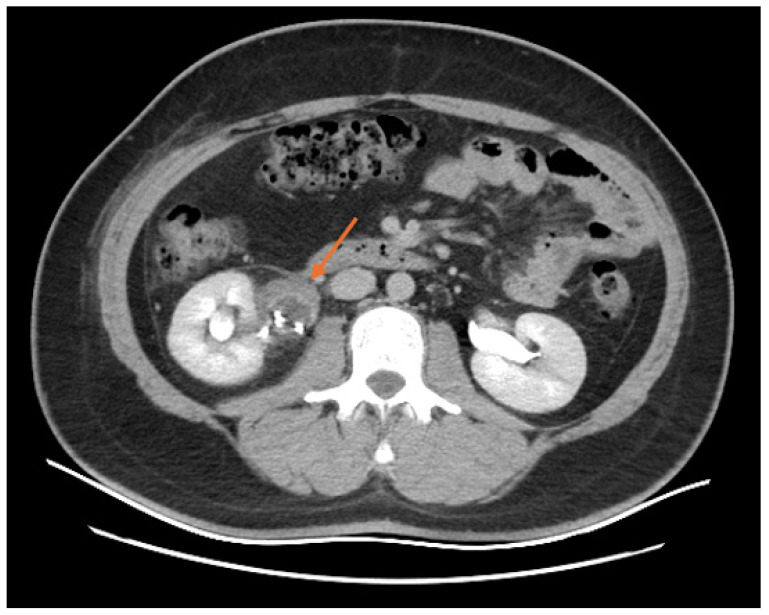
Thirty-days follow-up CT scan of the abdomen: ureteral stent (orange arrow).
